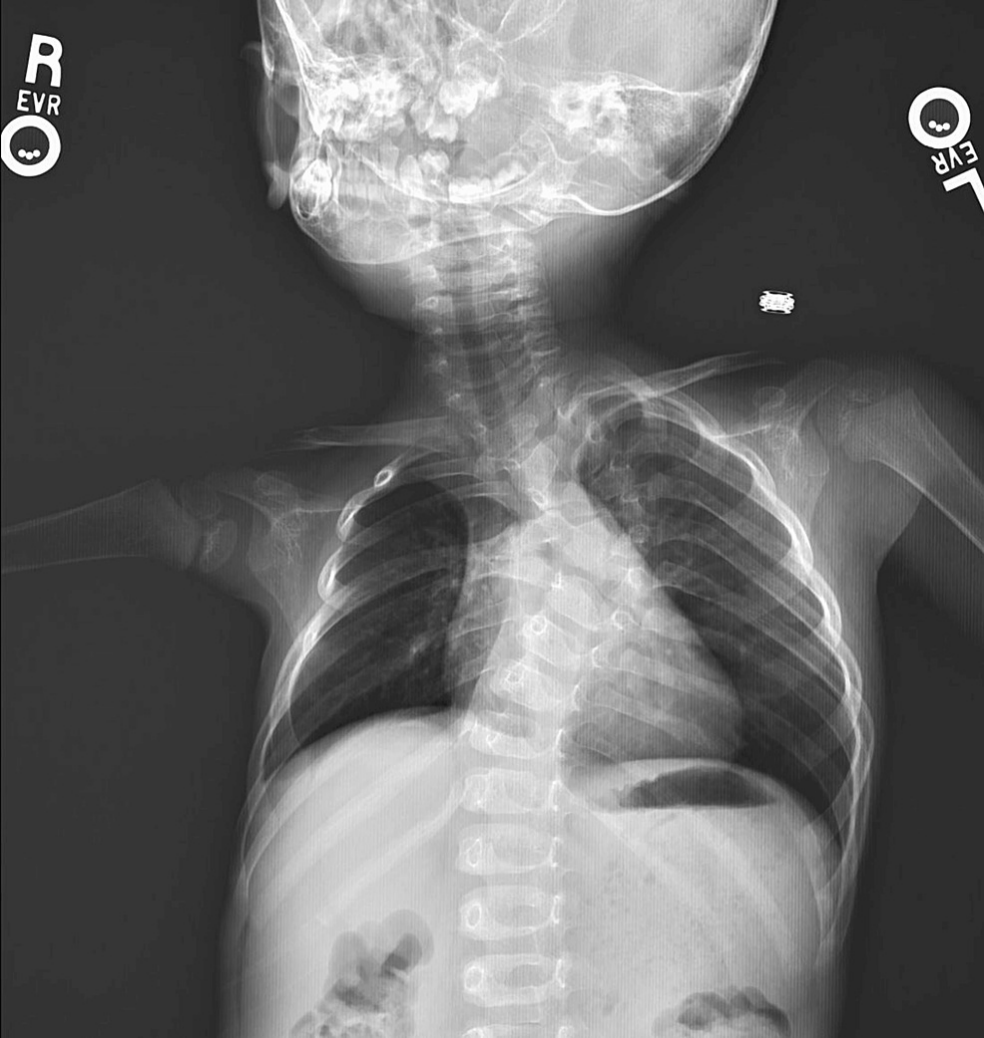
AP X-ray showing the patient's left convex upper thoracic congenital scoliosis AP: anteroposterior
